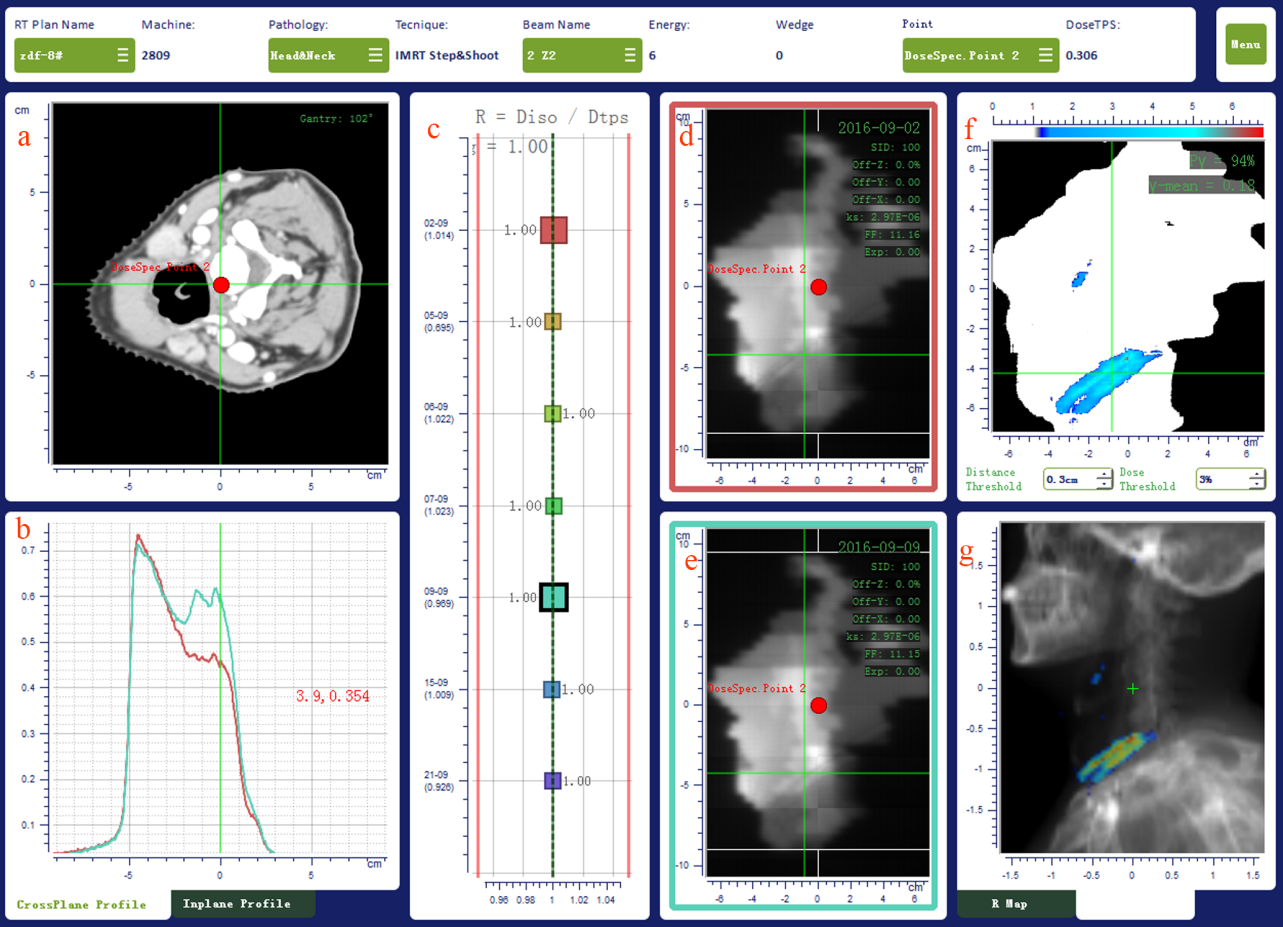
IVD results of a seven beams head and neck IMRT treatment as displayed on the main screen of SOFTDISO. The results for a 102° beam gantry angle of a head and neck IMRT treatment are displayed in (a) patient CT scan containing the isocentre point (red point), (b) EPID signal profiles, (c) R ratio on different days, (d) reference EPID image, (e) current EPID image, (f) map of points with *γ* > 1 (g) map of points with *γ* > 1 on the sagittal DRR image.
